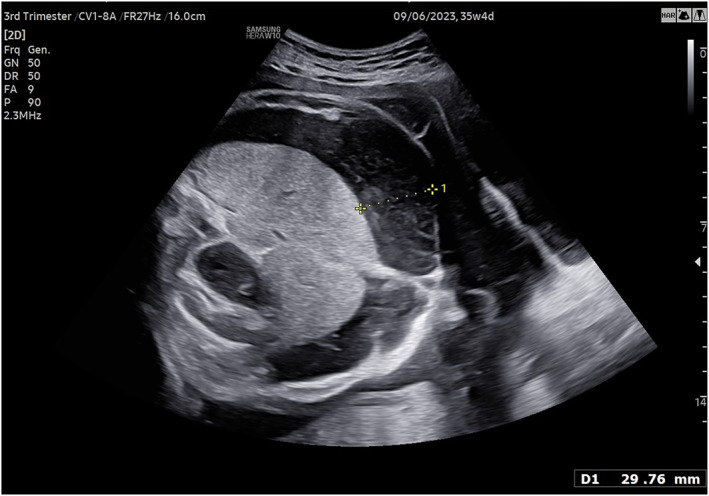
Ultrasound image (Transabdominal scan) of the abdominal ascites in a fetus with MPO.
